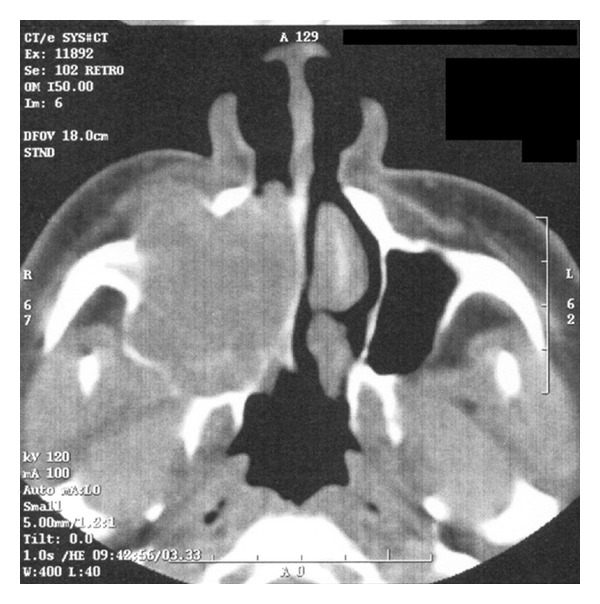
A preoperative CT showing a large lytic expansile, solid lesion extending from the nasopharynx area with obstruction of the ostiomeatal unit, and sphenoethmoidal recess into the lateral pharyngeal space, laterally to the parotid, without continuity with maxillary alveola and antrum.
